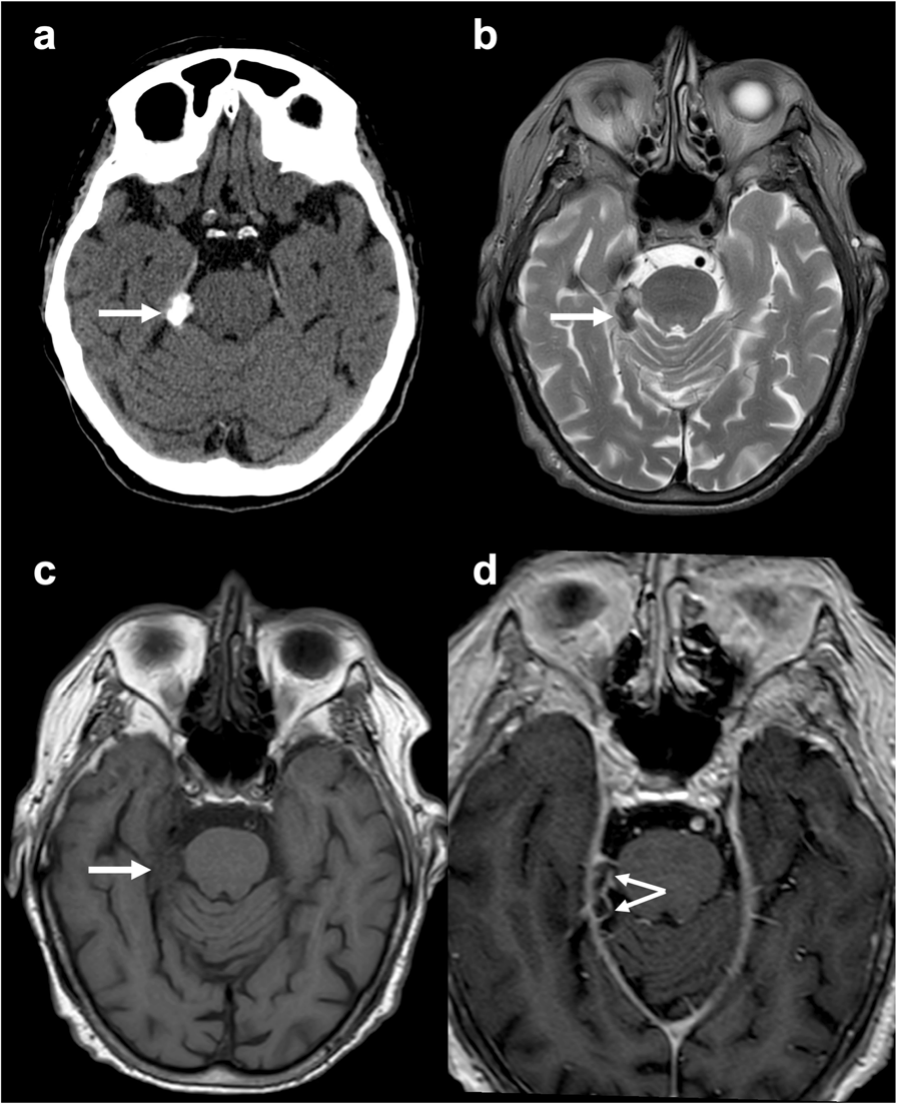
Teflon-induced calcified granuloma. Axial unenhanced CT (a), T2-weighted (b), unenhanced (c) and post-contrast (d) T1-weighted images depicting a calcified mass (arrow), with heterogeneous signal and rim enhancement
